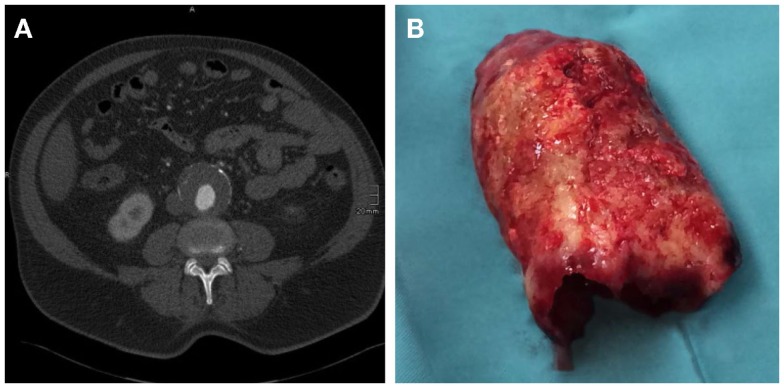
(A) CT axial image of an infrarenal AAA with intraluminal thrombus. (B) Corresponding, massive, intraluminal thrombus removed during open surgical repair.
